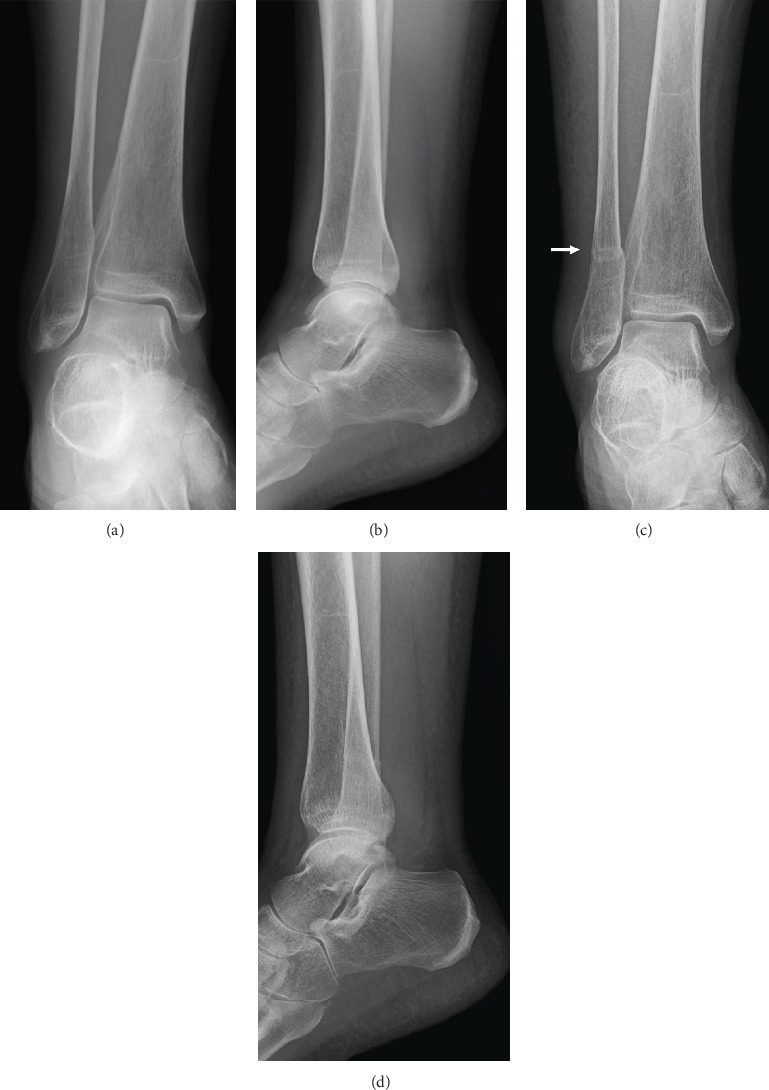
Bilateral radiograph of the right ankle joint immediately after presence of symptoms (a, b) and three weeks after that (c, d). Transverse liner fracture was obvious in (c) (arrow), but not in (a).
